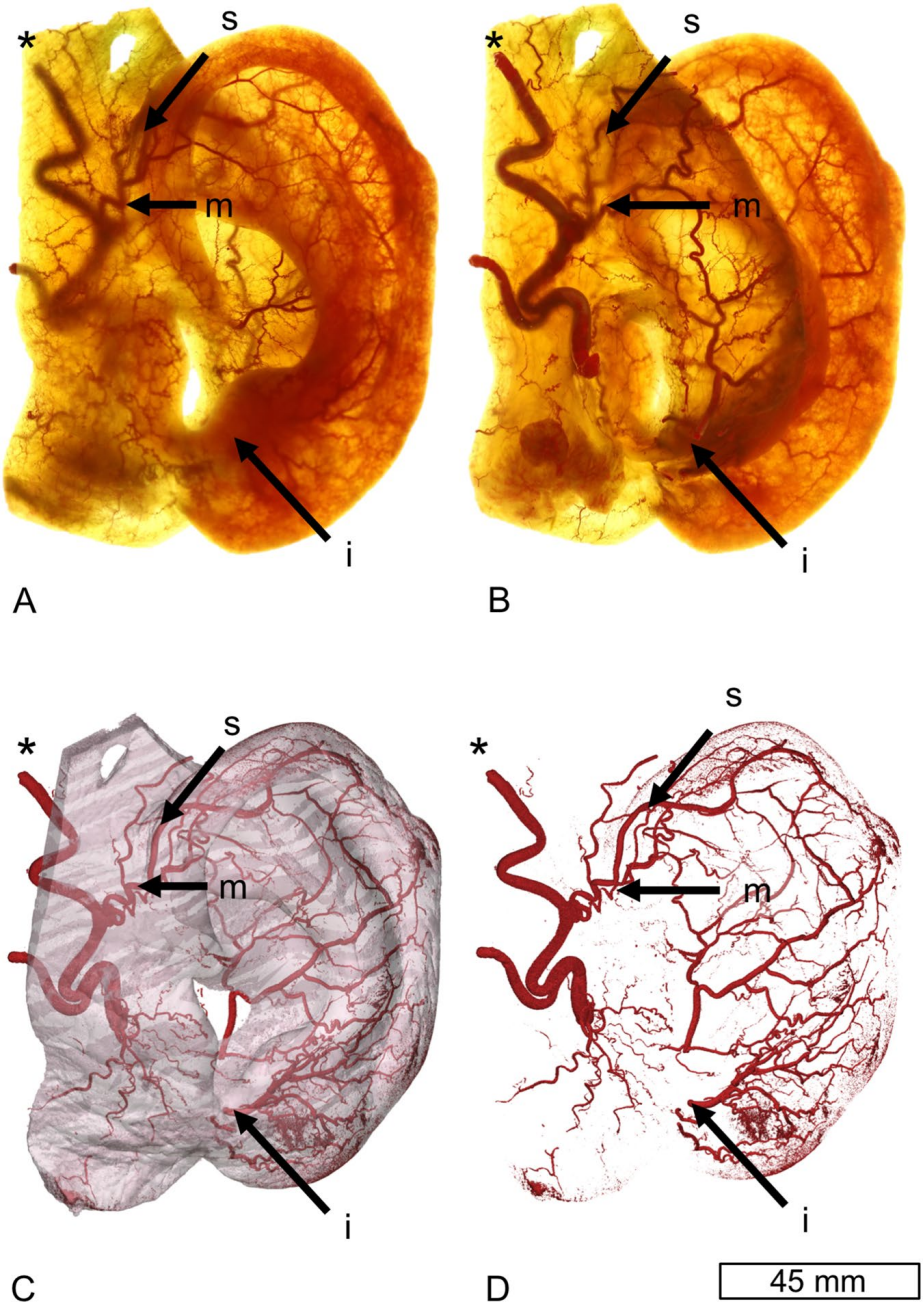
Lateral (A) and posterior view of a Spalteholz ear (B) showing auricular vascularity, and three-dimensional reconstruction using micro computed tomography in the surface topography (C) and isolated vascularity (D). s = superior anterior auricular artery, m = middle anterior auricular artery, i = inferior anterior auricular artery, *superficial temporal artery.
